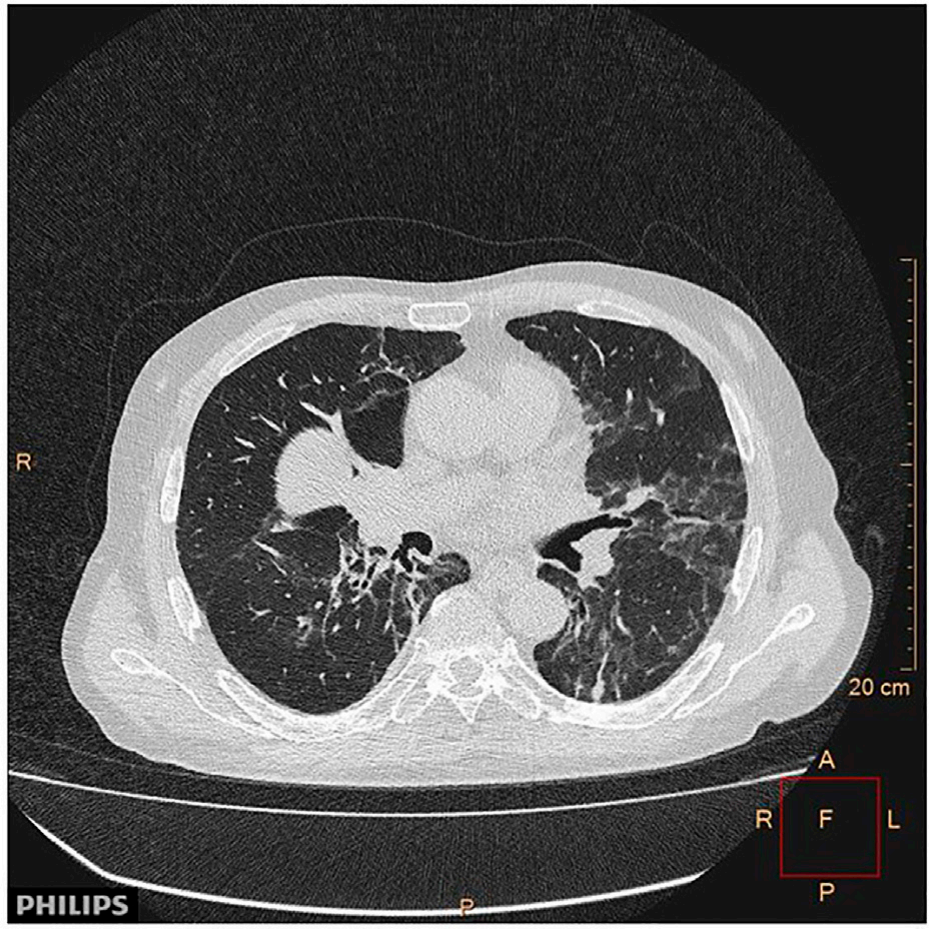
CT image of the pneumonitis caused by everolimus treatment of cancer patient. With permission of Hungarian Society of Clinical Oncology (5).
